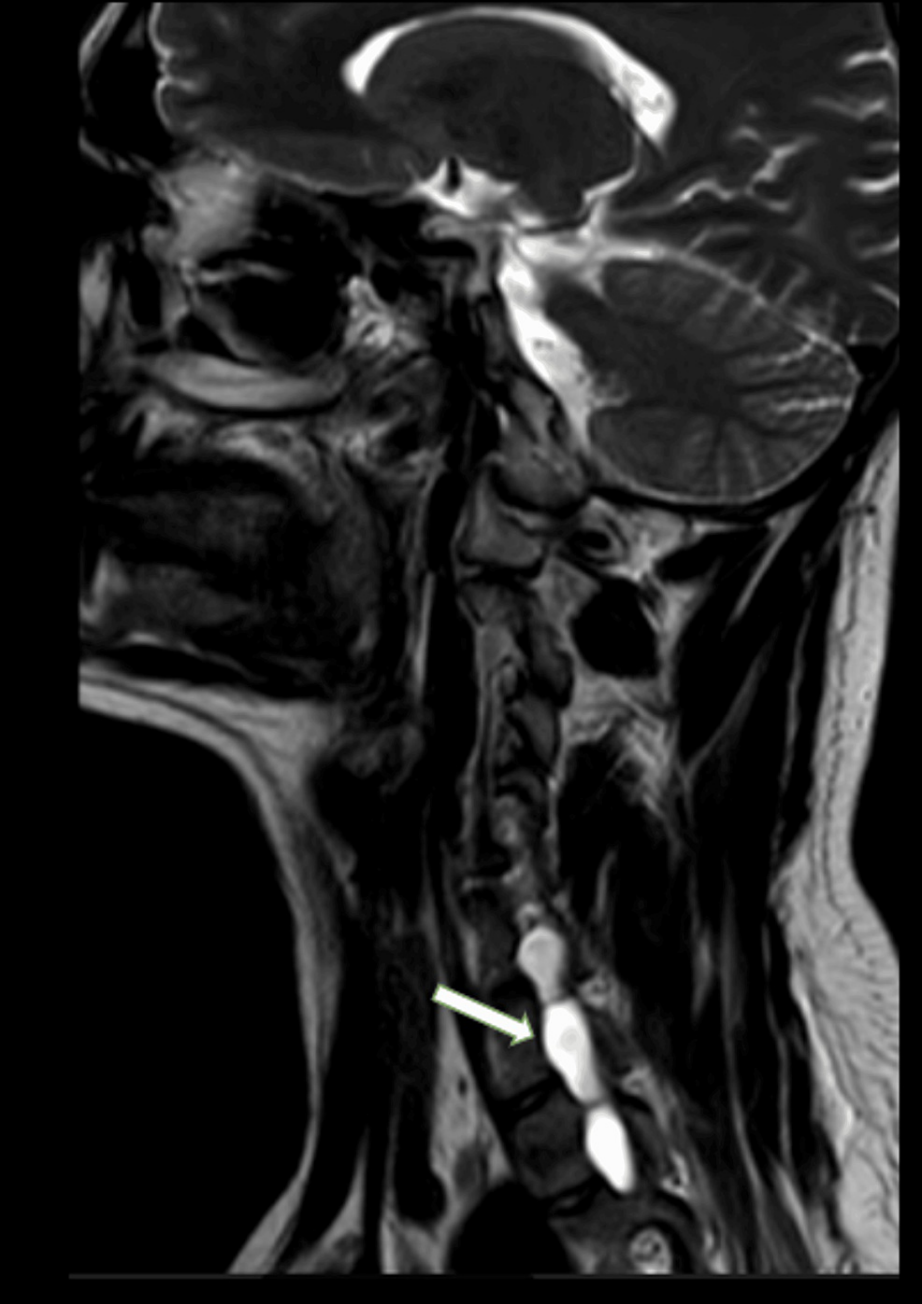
MRI-brachial plexus T2 right parasagittal section of case 1 MRI: Magnetic resonance imaging T2 right parasagittal section of the MRI-brachial plexus shows extradural, cystic formations that resemble cerebrospinal fluid collections within the spinal canal as indicated by the large thick white arrow. These collections are visible on both the dorsal and ventral aspects close to the spinal cord, extending through the right neural foramina at the levels of C6-C7, C7-T1, and T1-T2 vertebrae. Notably, these formations lack central neural elements and are bordered by smooth to lobulated margins.
